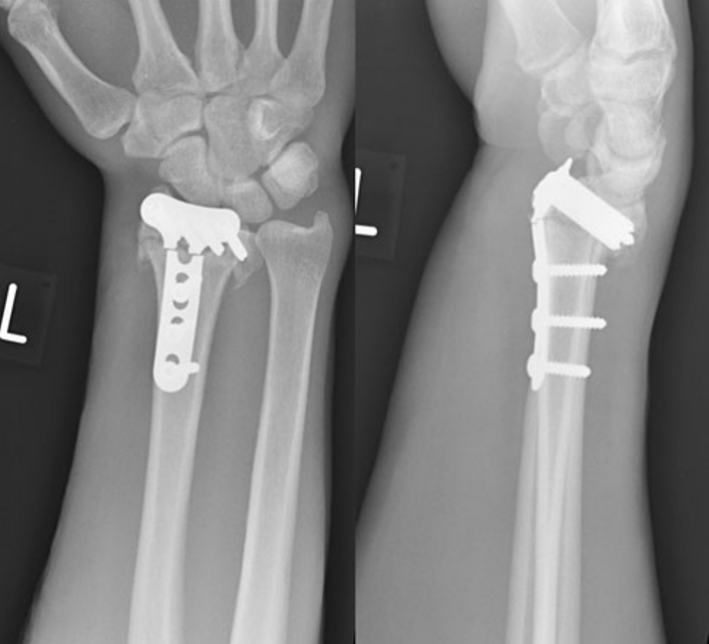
Radiographs at 6 weeks after the index procedure. Broken plate
